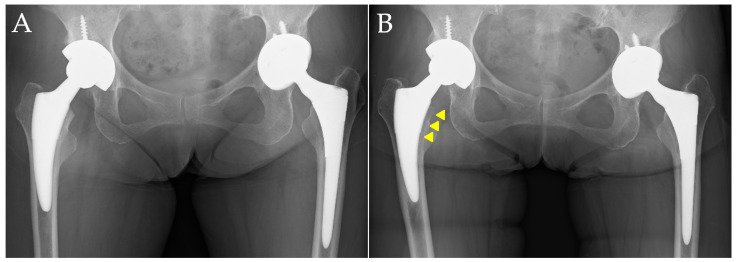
A representative case of advanced stress shielding. Case of a 73-year-old female patient. (A) Anteroposterior radiograph of the hip immediately after surgery; (B) anteroposterior radiograph one year postoperatively. The arrowheads indicate cortical bone thinning due to stress shielding, with progression of thinning observed up to the midsection of the stem.
